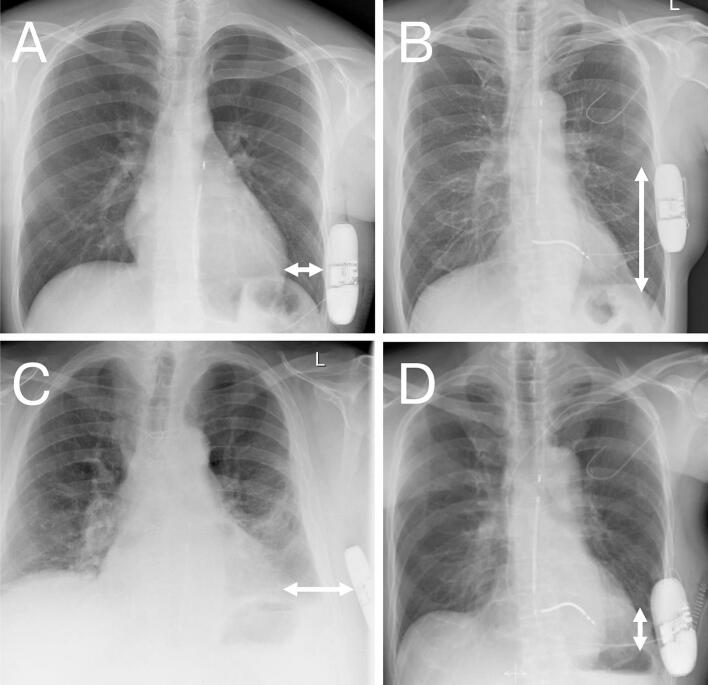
S-ICDs localized in the left axillary region (sub-serratus, intermuscular, and subcutaneous placement). Exemplary X-rays of S-ICD patients treated in our clinic. Depending on patient anatomy, varying distances between S-ICD and left ventricle occur: A) Normal-weight patient with a small distance between S-ICD and the heart in the left–right direction. B) Patient with a cranially implanted can and a resulting large craniocaudal distance between the S-ICD and the heart. C) Obese patient with a large distance between the S-ICD and the heart in the left–right direction. D) Patient with a caudal can placement and a resulting small craniocaudal distance between the S-ICD and the heart.
